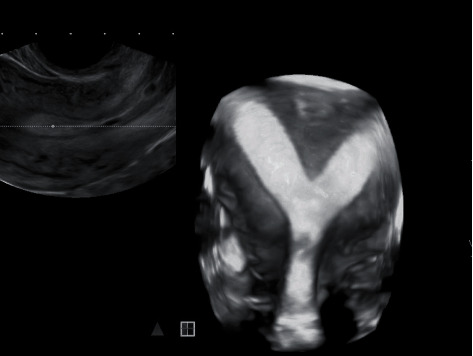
3D transvaginal ultrasound prior to conception demonstrates a large uterine septum. There is no fundal indentation seen.
